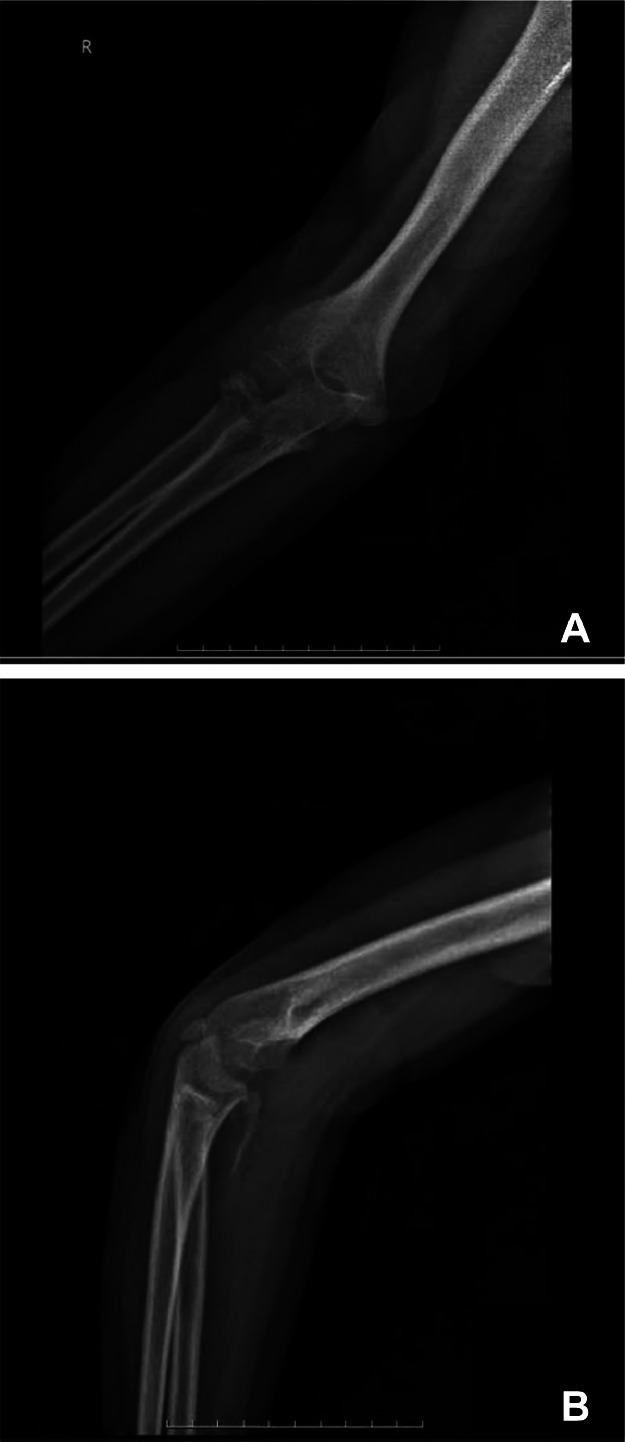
A: One-month follow-up anteroposterior radiograph of the right elbow Plain radiography (X-ray) in anteroposterior view, showing early healing of the radial head fracture following K-wire removal. Note the maintained joint reduction and improving fracture margins with early callus formation. B: One-month follow-up lateral radiograph of the right elbow Plain radiography (X-ray) in lateral view, demonstrating maintained reduction of the elbow joint after K-wire removal. The coronoid process and radial head show early signs of healing with no secondary displacement.
